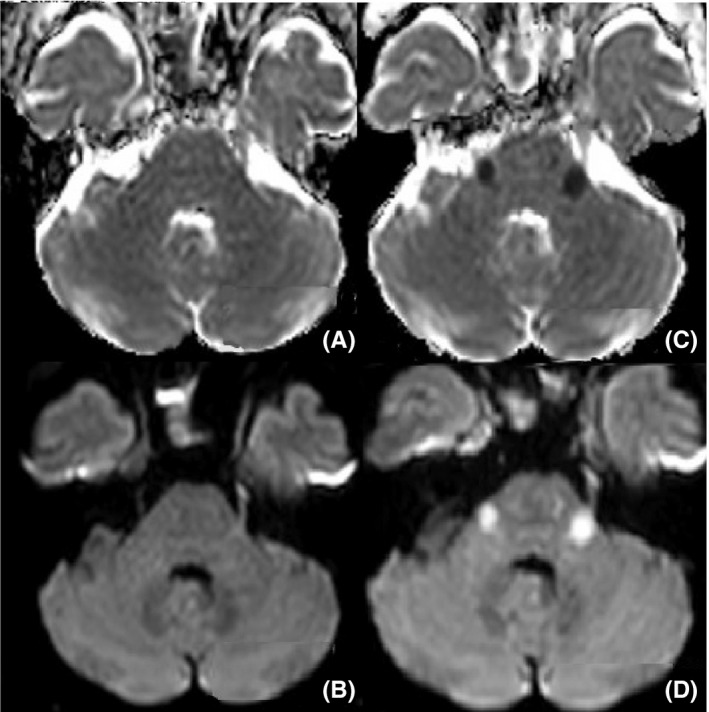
MRI sequences ADC and DWI done before (A; B) and after (C; D) 24 h that documented the appearance of two lesions in bilateral pontine sites, near the trigeminal nerve emergence
